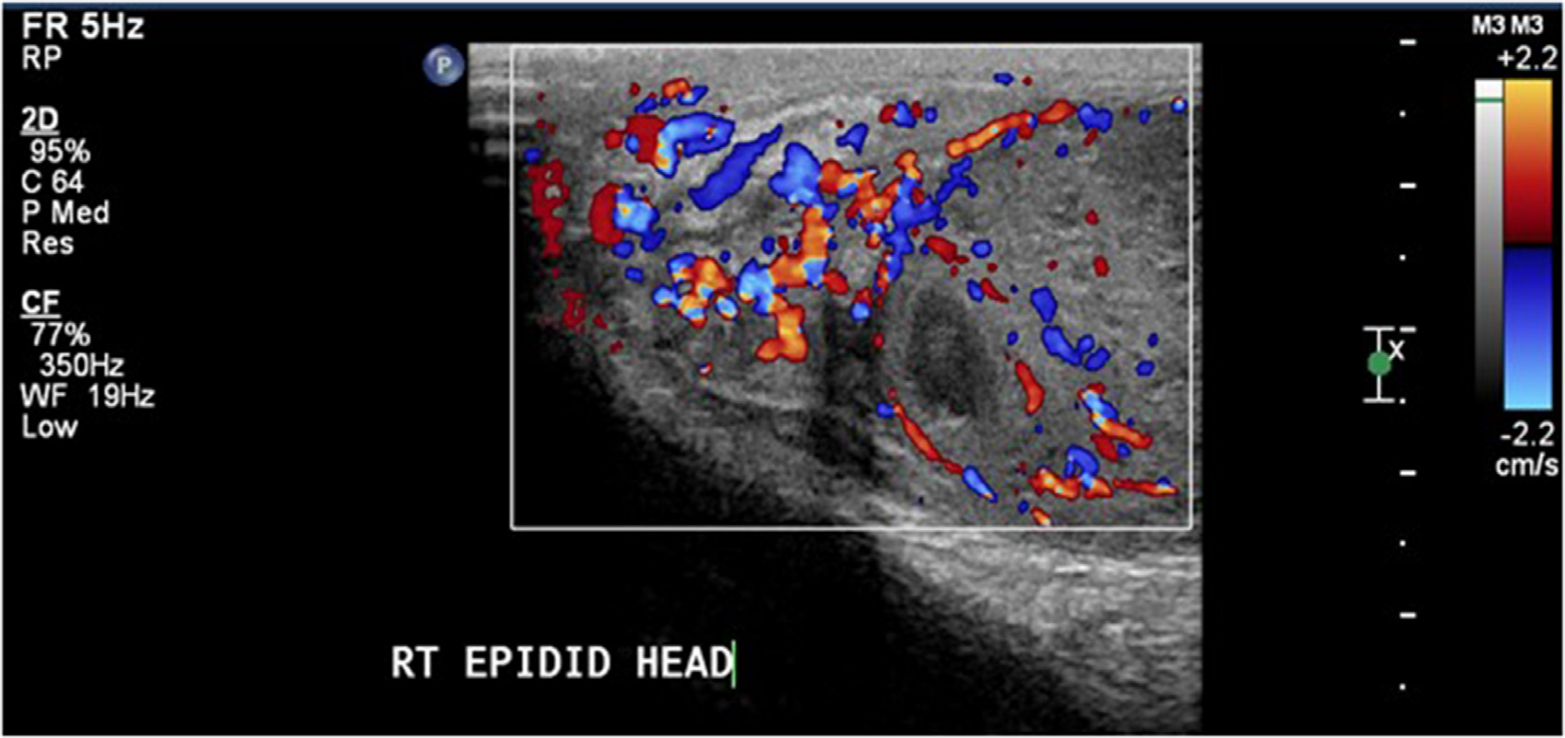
Doppler ultrasound image showing the severe hyperemia also involving the epididymis, in keeping with epidydimo-orchitis.
